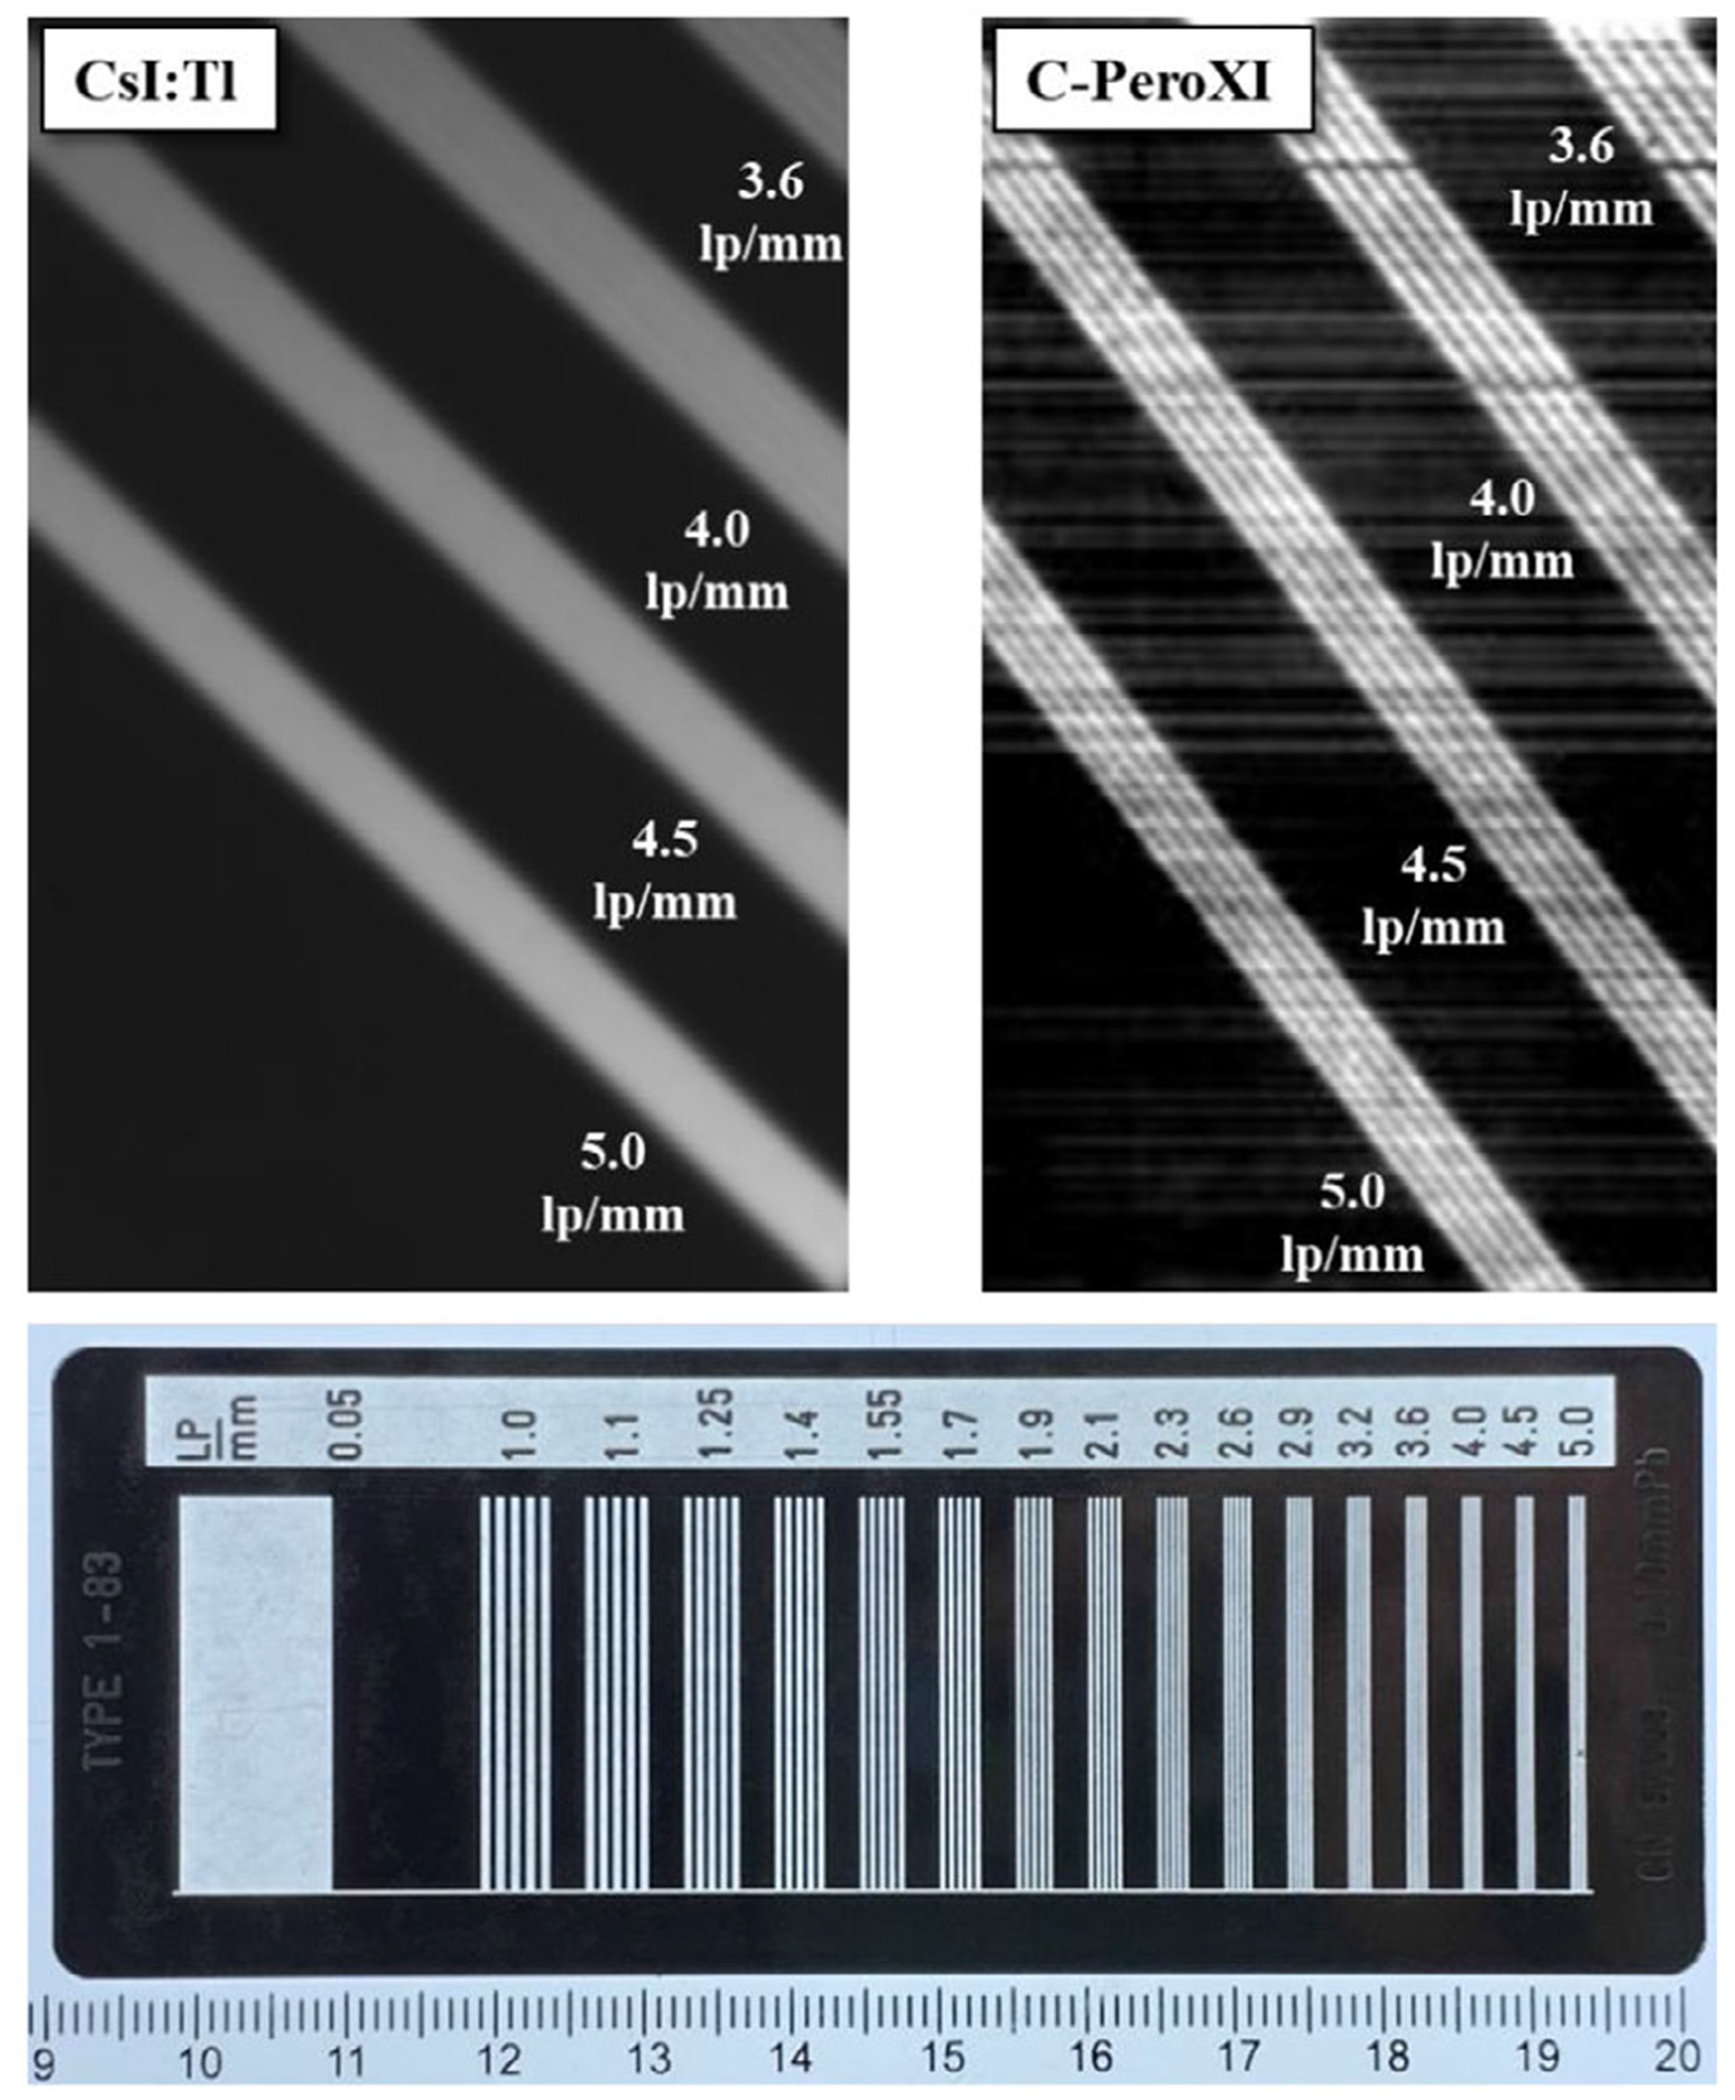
Comparison of raw X-ray images of a line pair pattern target obtained using different detectors of same detection efficiencies. Top left: Image taken with a CsI:Tl scintillator-based indirect detector. Top right: Image taken with direct conversion C-PeroXI with 50 keV X-rays. The C-PeroXI demonstrates superior spatial resolution, clearly resolving line pairs up to 5.0 lp/mm, while the CsI:Tl detector shows blurring at higher spatial frequencies. Bottom: Photograph of the actual line pair pattern target used, showing spatial frequency markings from 0.05 to 5.0 lp/mm. The enhanced contrast and sharpness of the C-FPXI image highlight the advantages of direct conversion perovskite detectors for high-resolution X-ray imaging applications.
